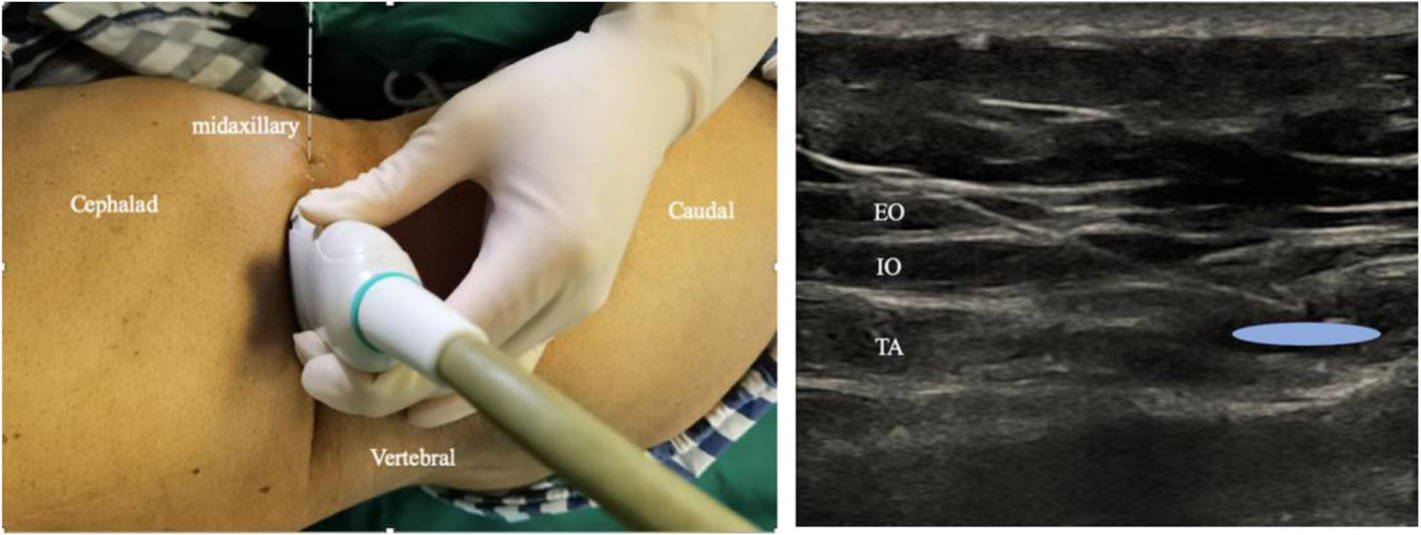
Posterior approach of transversus abdominis plane (TAP) block. Note: a The patient was kept in semi-lateral position, the probe position and needle trajectory were displayed. The probe is placed posterior to the midaxillary line between the costal margin and the iliac crest. The needle is inserted in plane. b Corresponding ultrasound images. Posterior approach located in the end of transversus abdominis plane where TAP transmigrate into aponeurosis. The injection site is at the TAP between internal oblique and transversus abdominis posterior to the midaxillary line and near the aponeurosis. White dashed line: needle trajectory. Light blue area: the deposition site of local anesthetic. TA: transversus abdominis; IO: internal oblique; EO: external oblique
